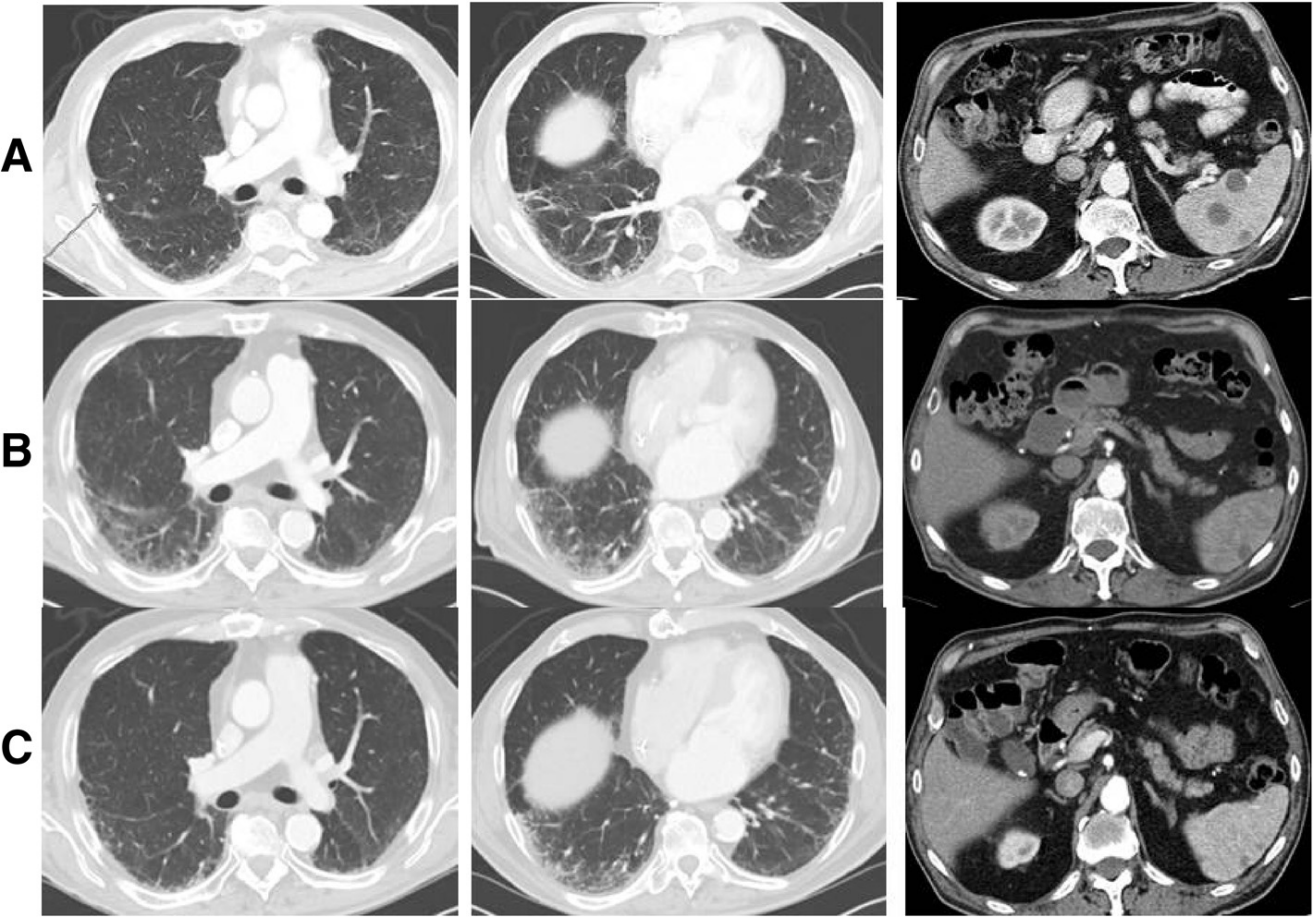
Pre- and post-treatment CT images for patient 2. A. Pre-treatment CT images of metastatic lung nodules and splenic metastases. B. Post-treatment CT images of resolving lung and splenic metastases. C. Twenty month post-treatment CT images of resolved lung and splenic metastases.
